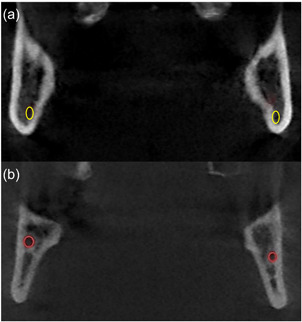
Coronal view of the mandible in cone‐beam computed tomogram image. Lingual (a) and buccal (b) mandibular canal is marked in red.
